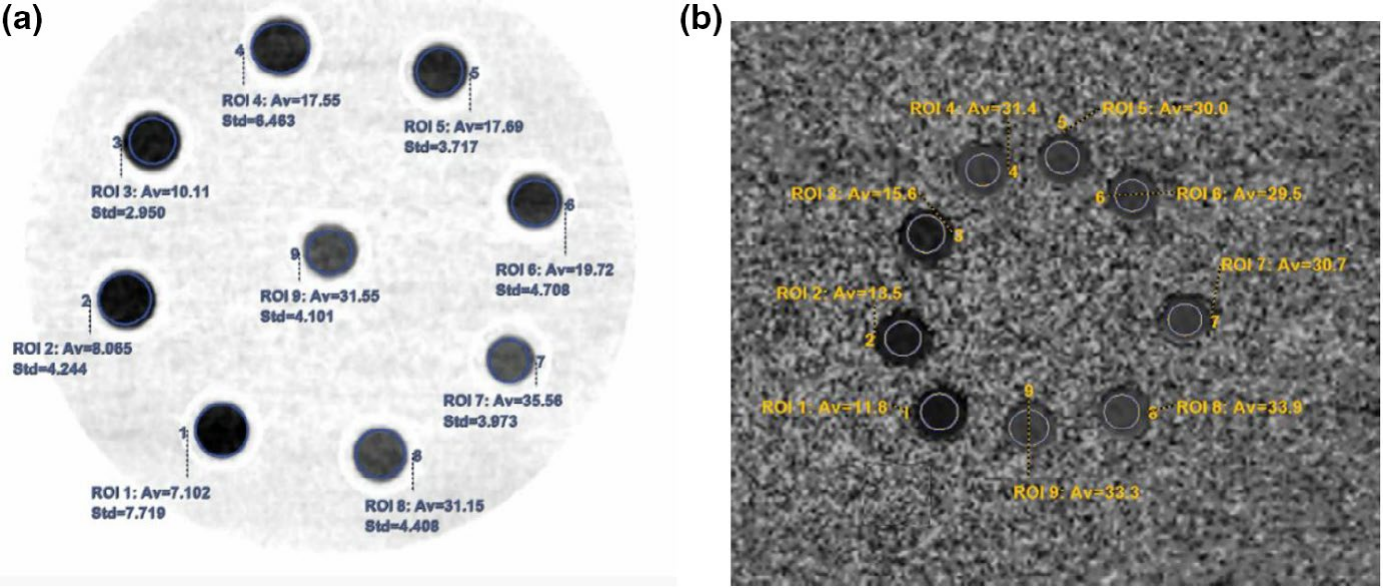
The exact values in nine ROI tubes by CT and MR fat fraction images. (a) Fat fraction image from MMD; (b) Fat fraction image from IDEAL‐IQ. The numbers correspond to the nine tubes shown in Tables 1 and 2. IDEAL‐IQ, Iterative Decomposition of water and fat with Echo Asymmetry and Least squares estimation; MMD, multi‐material decomposition; MR, magnetic resonance; ROI, region of interest
